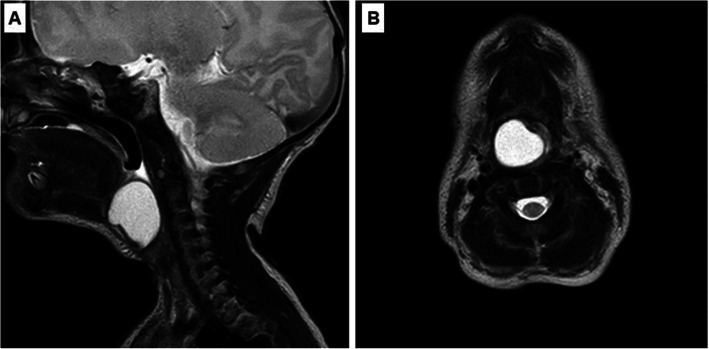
Preoperative MRI. A: Sagittal contrast MRI image shows well-circumscribed, thin-walled fluid attenuation mass. B: Axial contrast MRI image reveals the cystic mass at the level of the glottis
